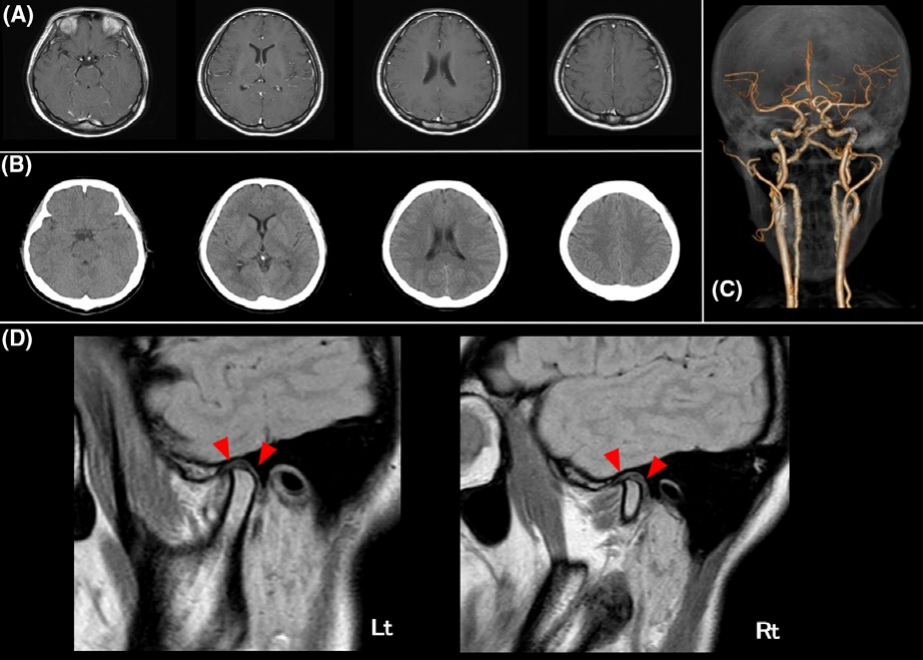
A, Contrast‐enhanced MRI showed no contrast effect in the meninges or brain parenchyma. B, Head computed tomography showed no cerebral hemorrhage. C, Computed tomography angiography did not reveal vertebral artery dissection or cerebral aneurysm. D, Proton density weighted MRI showed a narrow temporomandibular joint head and thinning of the temporomandibular disk (red arrow head) but did not reveal a temporomandibular disorder
